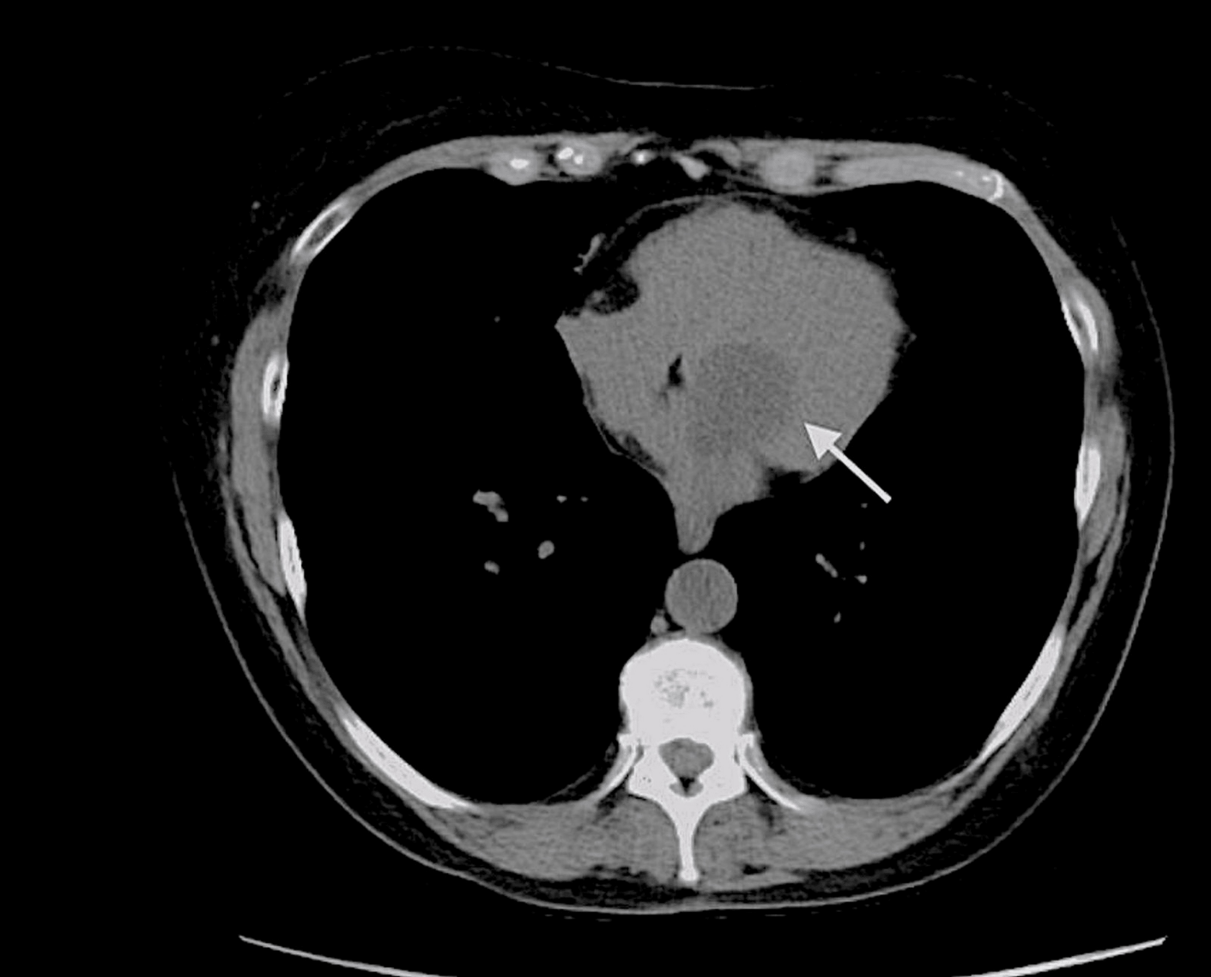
CT of mass near the left atrium
